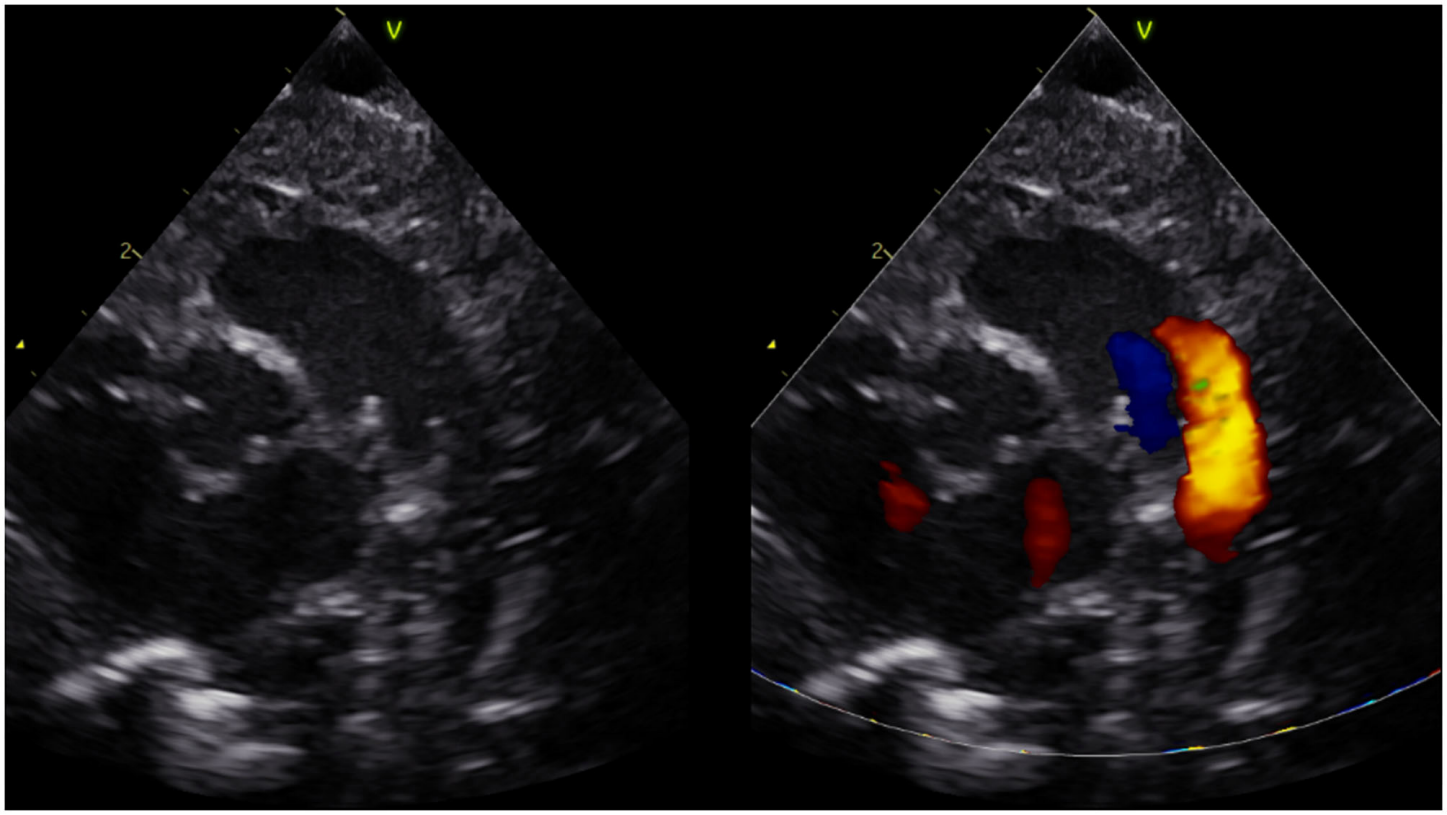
Short axis parasternal view of ductus arteriosus and pulmonary arteries (three vessel view).
